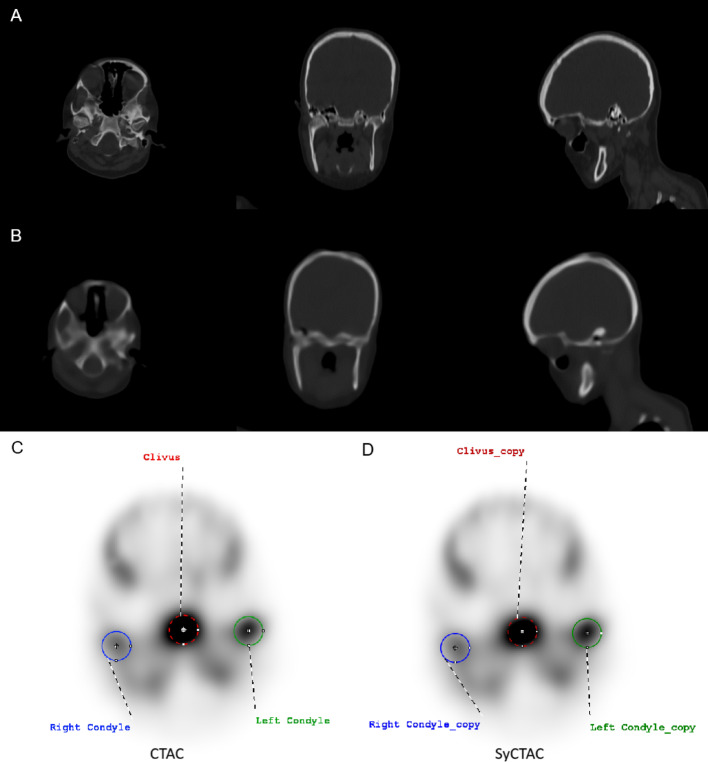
Axial, coronal and sagittal slices of CT (A) and SyCT (B) images. Axial slices of the CTAC (C) and SyCTAC (D) images with spherical VOIs (diameter: 2 cm) placed over both condyles and the clivus
